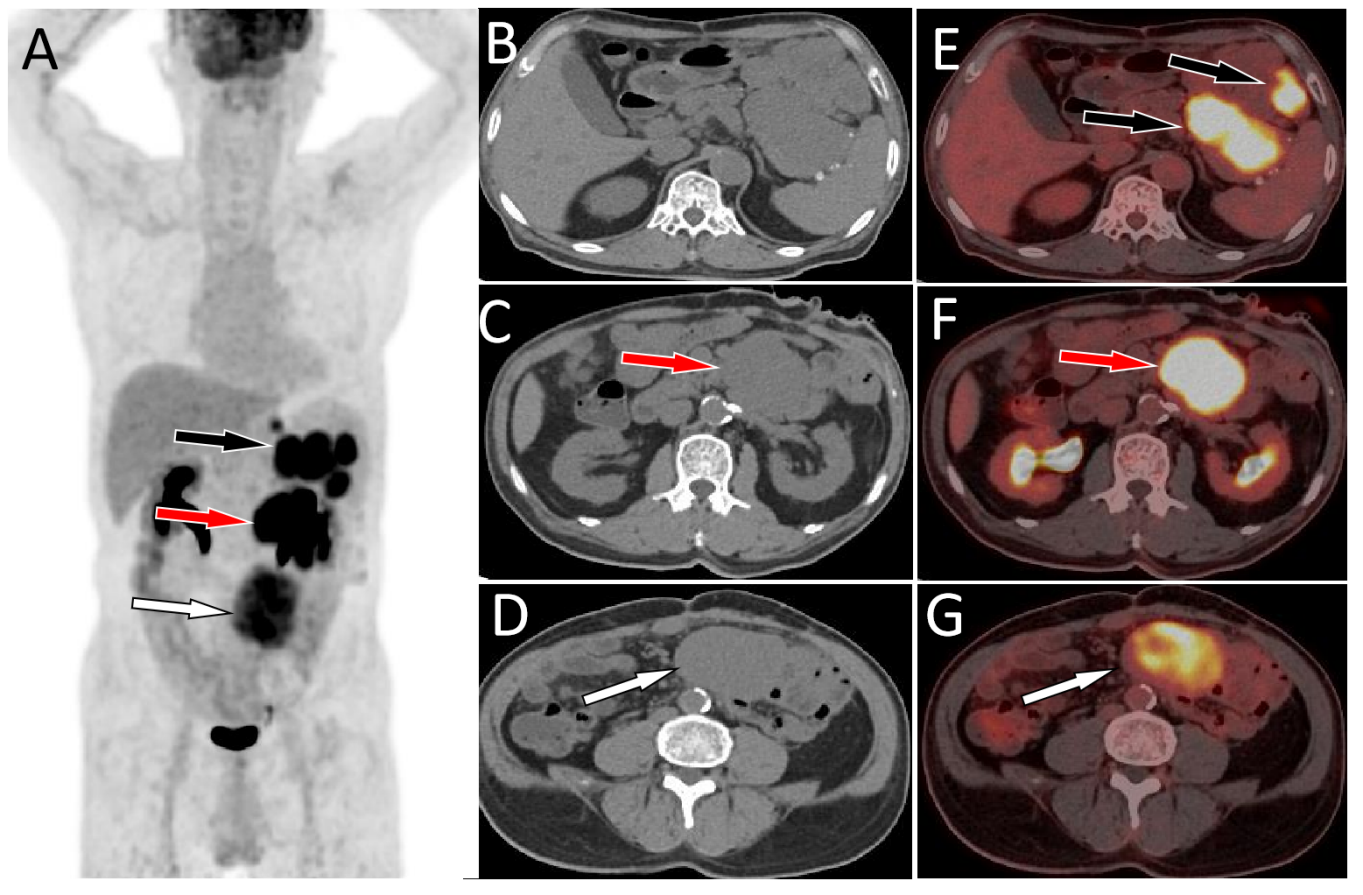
MIP image (A) acquired 60 min after injection showed that large intensity uptake in the left upper abdomen with SUVmax of 13.2 (black arrow), 14.6 (red arrow) and moderate radioactive uptake overlapping the spine with SUVmax of 9.1 (white arrow). On axial CT (B-D) and PET/CT fusion (E-G) images, the mass with high radioactivity uptake was located in the spleen-gastric space (black arrow), left retroperitoneum (red arrow), and right retroperitoneum (white arrow). Notes: MIP, maximum intensity projection; CT, computed tomography; PET, positron emission tomography; ^18^F-FDG, ^18^F-labeled fluoro-2-deoxyglucose; SUVmax, maximum standard uptake value.
